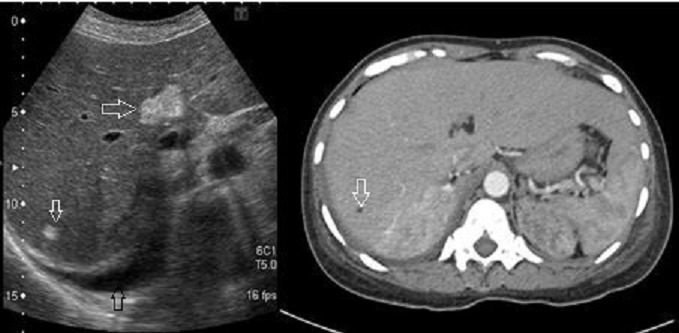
Ultrasound image shows hepatic lipomas appearing as hyperechoic lesions (white arrows). Right pleural effusion is also noted (black arrow). Corresponding CT section show fat attenuation in hepatic lesions (white arrow, right image)
